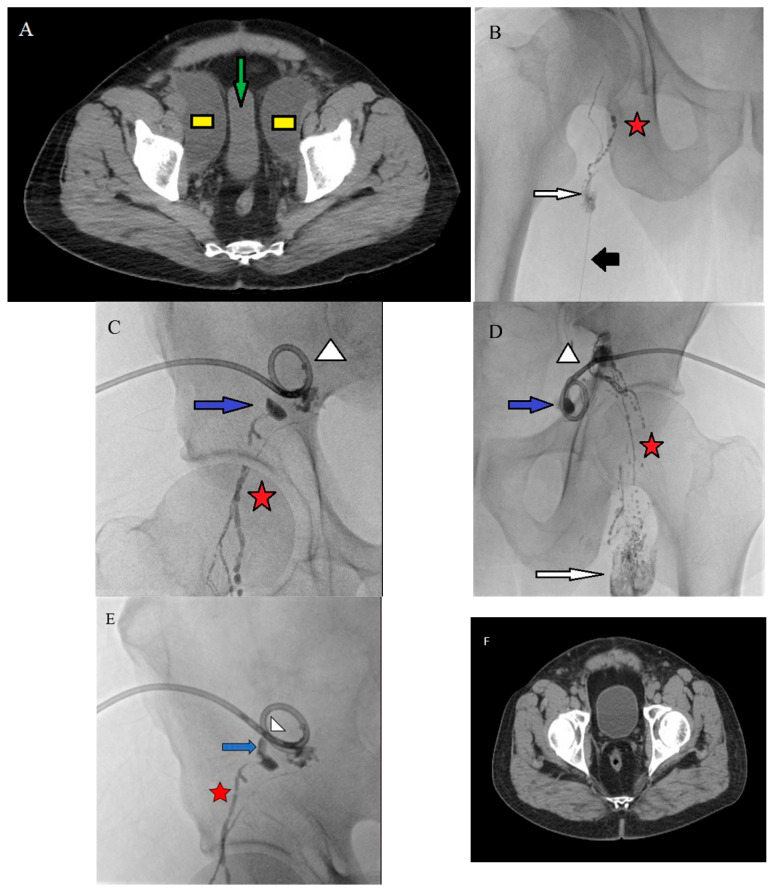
(A) Cystic collections consistent with bilateral pelvic lymphoceles (yellow boxes) and compression-related transverse narrowing of the urinary bladder (green arrow). (B) A 24G needle puncturing an inguinal lymph node (black arrow), opacification of medullary lymphatic ducts within the lymph node using Lipiodol (white arrow), and Lipiodol-opacified lymphatic channels distal to the node (red asterisk). (C) Drainage catheter within the right lateral pelvic collection (white arrow). Accumulated Lipiodol within the cystic cavity confirms the lesion as a lymphocele, with Lipiodol-opacified lymphatic ducts also visible (red asterisk). (D) Drainage catheter within the left lateral pelvic collection (white arrowhead), Lipiodol contrast material pooling within the cavity (blue arrow), Lipiodol-opacified medullary lymphatic channels of the left inguinal lymph node (white arrow), and Lipiodol-opacified lymphatic ducts distal to the node (red asterisk). (E) After visualization of lymphatic ductal leakage and opacification of the lymphocele cavity with Lipiodol, a Lipiodol–NBCA mixture was injected through the same lymph node access. The dense embolic material is seen to reach the injured lymphatic leak site (blue arrow) via the lymphatic duct (red asterisk) and to fill the lymphatic cavity (white arrowhead). (F) Post-treatment follow-up CT image demonstrating complete resolution of the lymphatic collections.
